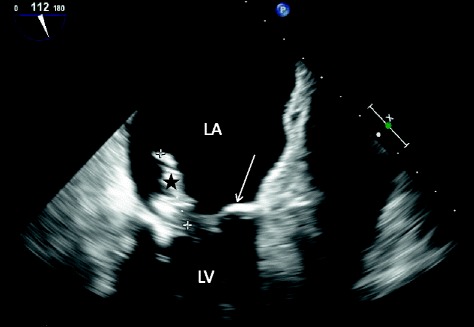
Transesophageal echocardiogram of infected native mitral valve. Echocardiography visualized a 28.6 mm multilobulated vegetation (black star), attached to the native mitral valve (arrow). Left atrium (LA). Left ventricle (LV). The 3D color Doppler (not shown) revealed a severe valve dysfunction with mitral regurgitation
